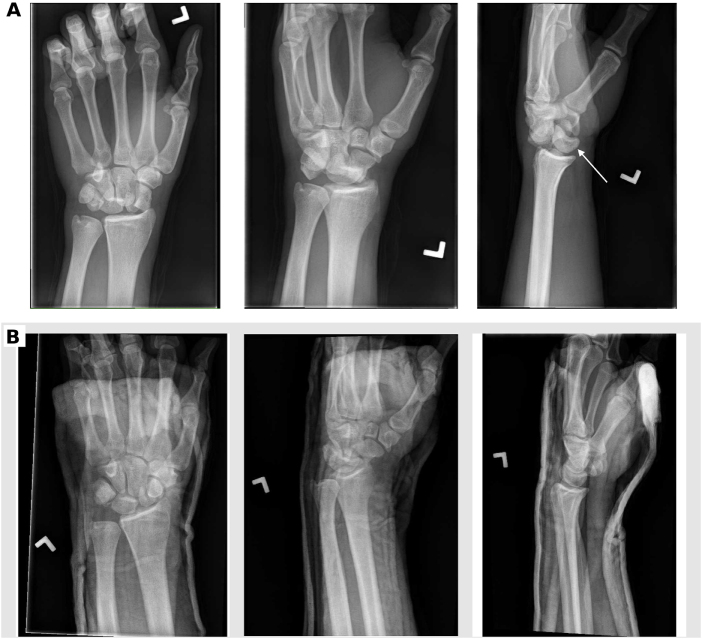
(A) (Case 1, initial injury – pre-reduction): Three-view radiographs of the left wrist demonstrate a trans-scaphoid perilunate fracture-dislocation, with dorsal displacement of the capitate/carpus relative to the lunate on the lateral view, consistent with a perilunate injury pattern. (B) (Case 1, post-reduction – emergency department): Three-view radiographs of the left wrist demonstrate improved carpal alignment after closed reduction. Residual malalignment is present, with radial translation/subluxation of the capitate relative to the lunate, as well as an associated scaphoid fracture and triquetral fracture/avulsion.
